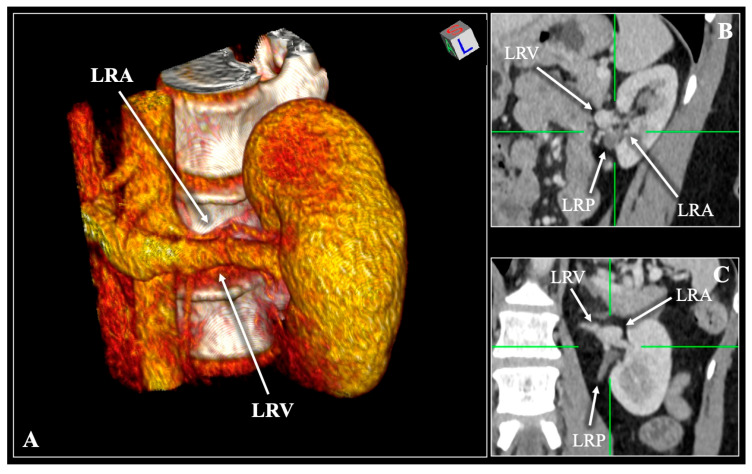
Contrast-enhanced computed tomography (CT) scan of a 57-year-old female patient demonstrating the Vein (V)—Pelvis (P)—Artery (A). Three-dimensional reconstruction (A) and multiplanar reconstruction with sagittal (B) and coronal (C) slices. Note the renal pelvis (LRP) interfering between the left renal vein (LRV) and artery (LRA) in sagittal and coronal reconstruction (B,C). R—right, L—left, S—superior, I—inferior.
